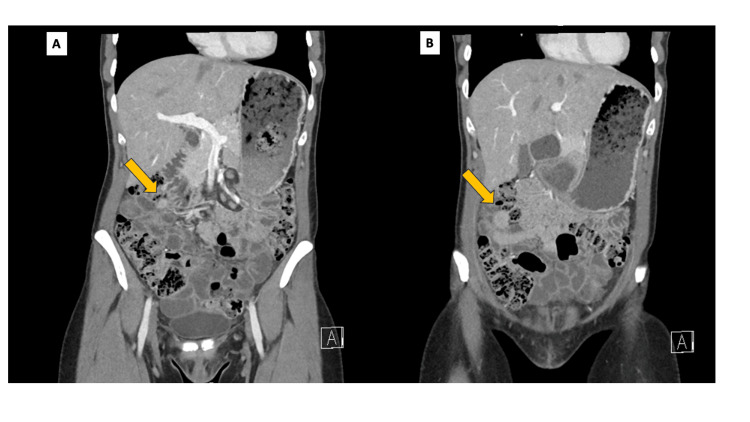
Case 2: CT images of ectopic pancreas. (A) Coronal CT image obtained after recovery from the initial episode of small bowel obstruction and prior to definitive surgical intervention (preoperative CT), demonstrating small bowel loops without a clearly definable focal mass at the time of prospective interpretation. (B) Coronal CT reconstruction again failing to clearly characterise a discrete lesion. On retrospective review, a subtle focal concentric configuration (orange arrow) may correspond to the site of intussusception identified intraoperatively. CT: computed tomography
